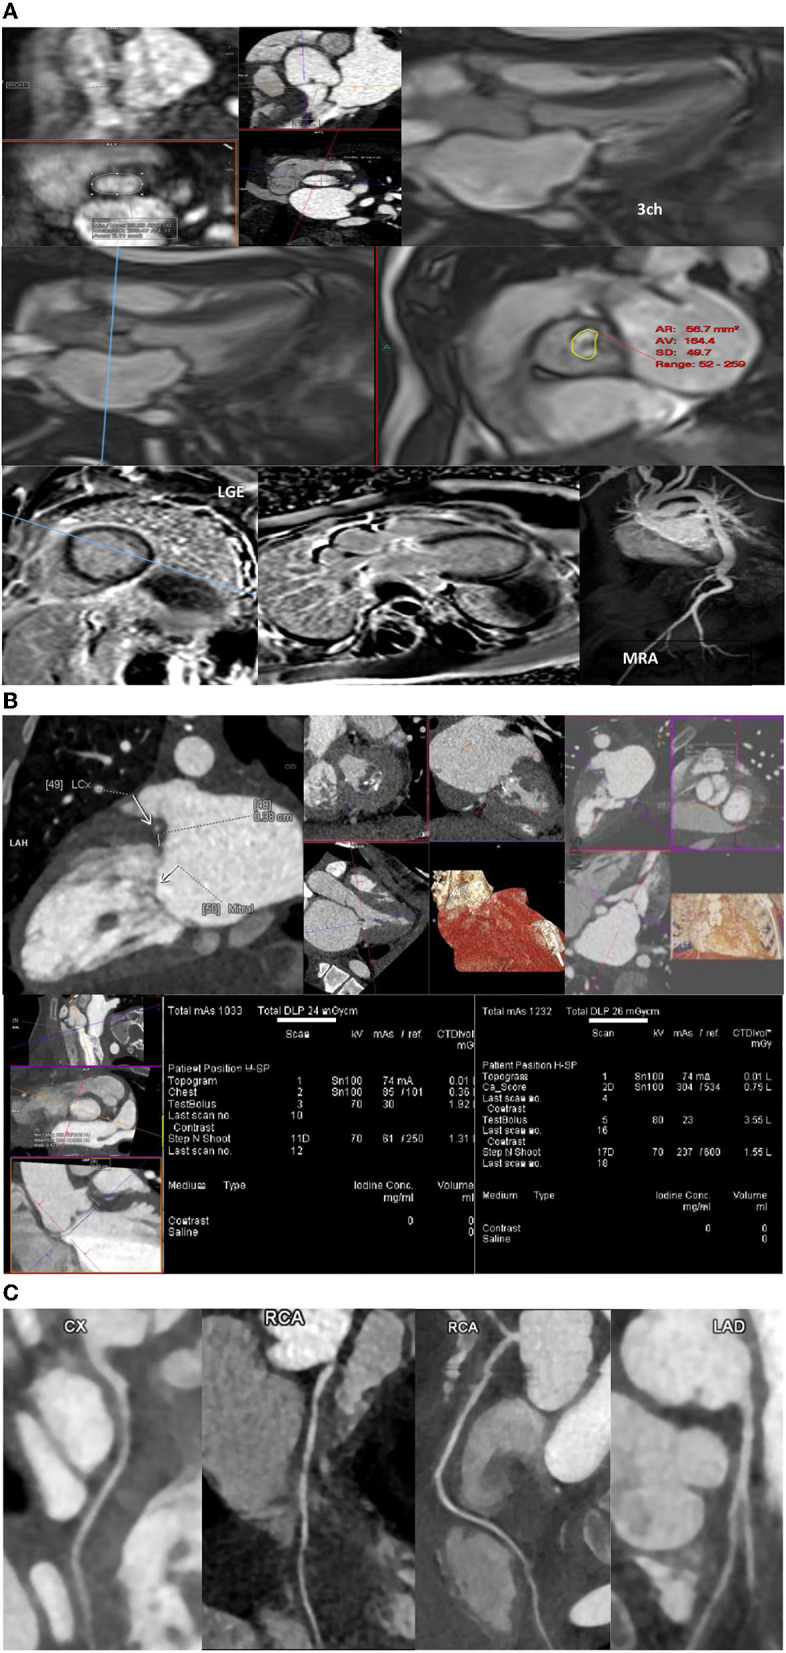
(A–C) Magnetic resonance imaging, computed tomography, and coronary magnetic resonance imaging in MPS patients.
